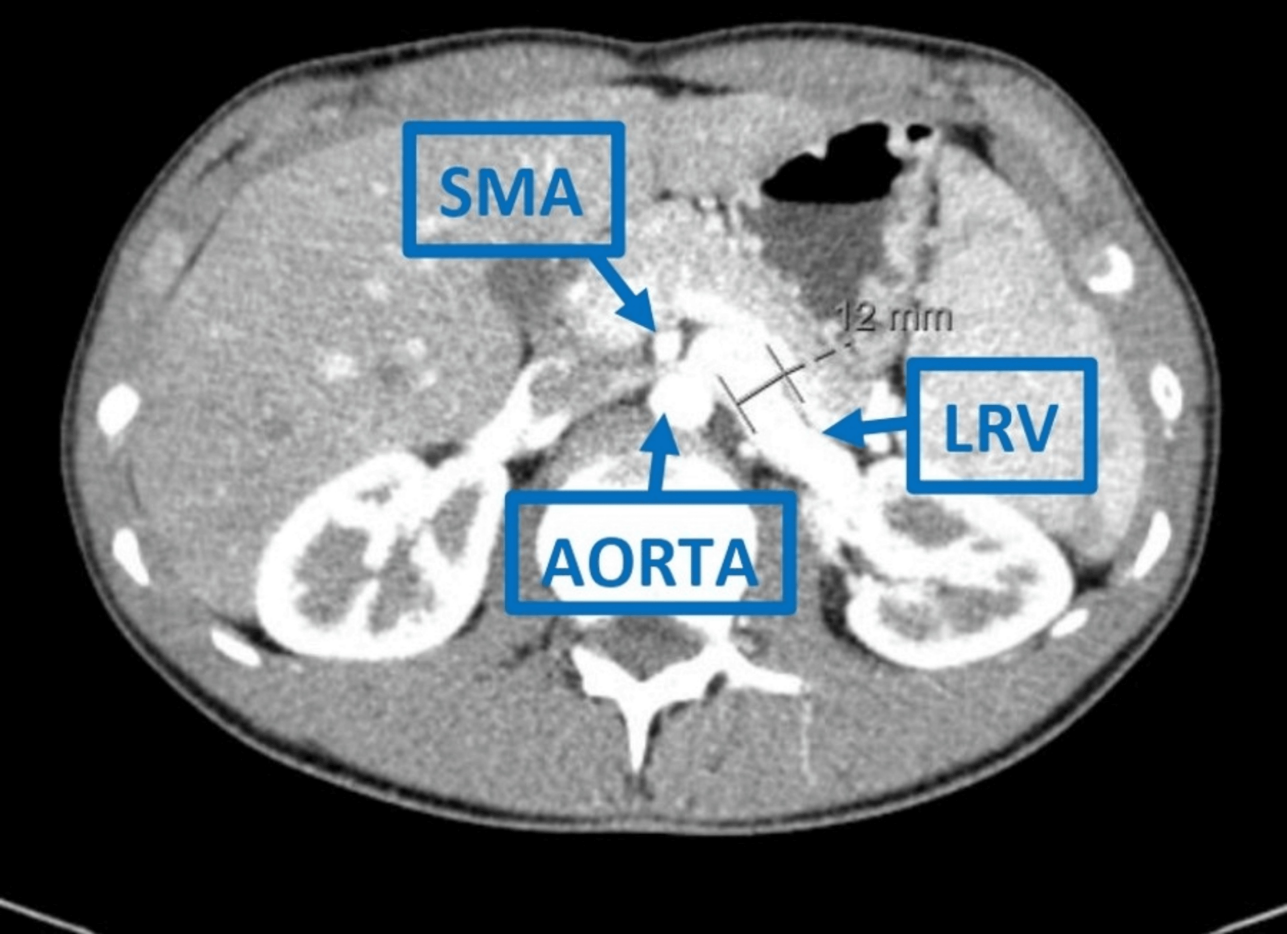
Axial Plane Computed Tomography (CT) Scan Axial plane abdominal CT scan demonstrating compression of the left renal vein (LRV) between the superior mesenteric artery (SMA) and the aorta, resulting in proximal LRV dilation. Findings are consistent with nutcracker syndrome.
